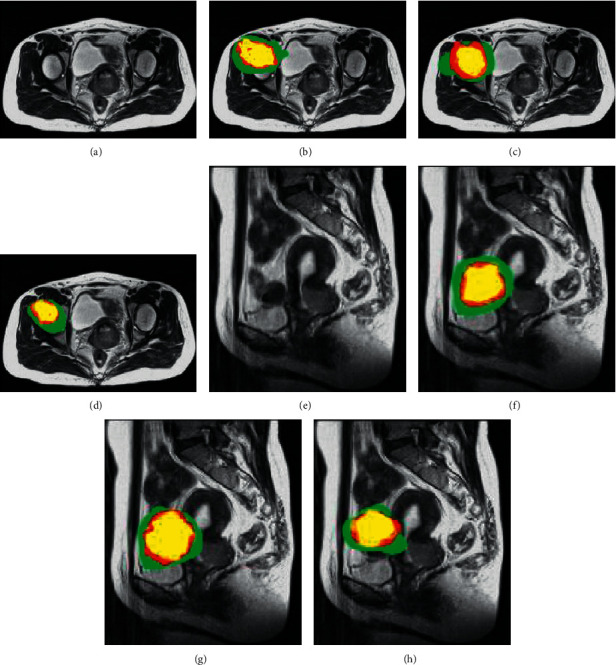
Visual comparison results of multimodal MRI images in the experiment. (*Note*. A and E are the transverse and sagittal images of multimodal MRI in patients under normal conditions. B and F are the prediction results of cervical cancer lesions in transverse and sagittal planes delineated by doctors. C and G are the prediction results of cervical cancer lesions in transverse and sagittal positions of the traditional CNN algorithm. D is the prediction of cervical cancer lesions in transverse and sagittal positions by the artificial intelligence 3D-CNN algorithm).
